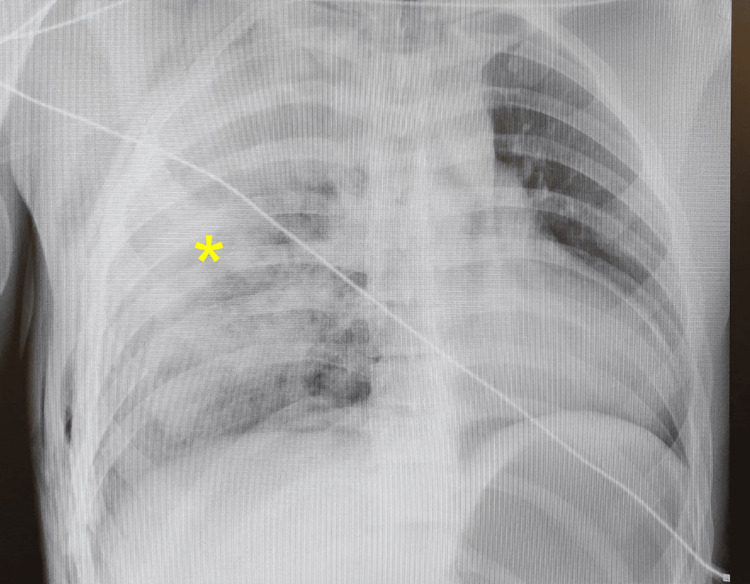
Chest X-ray revealing a right unilateral whiteout and subcutaneous emphysema involving the right thoracic wall resulting from a right hemopneumothorax (yellow asterisk).
